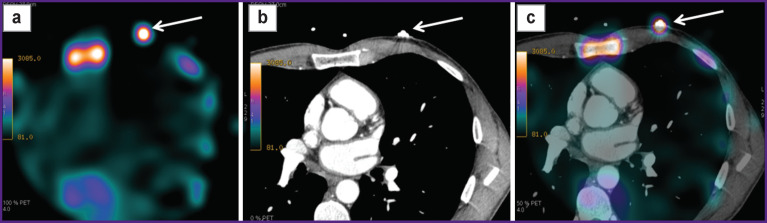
An example of combining scintigraphic and X-ray images using the surface markers: (a) scintigraphic image (axial plane), the arrow indicates the location of the radioactive marker; (b) X-ray tomographic image (axial plane), the arrow indicates the location of the radiocontrast marker; (c) hybrid SPECT/CT image, the arrow indicates the alignment of the both markers
